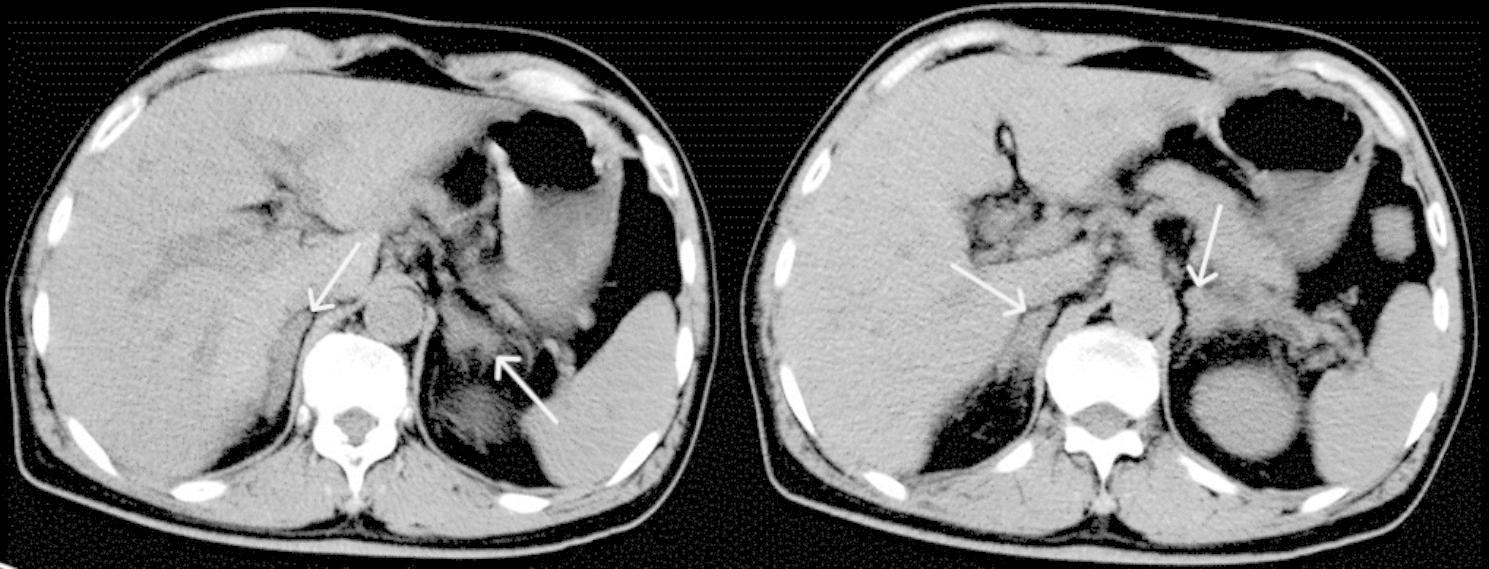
Abdominal CT of the patient showing enlarged adrenal glands
